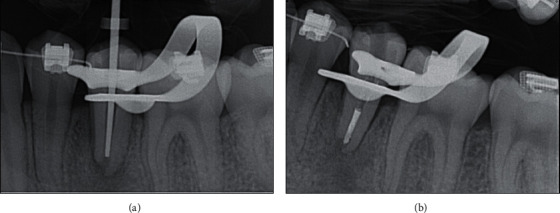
Obturation radiographs. (a) Periapical radiograph of endodontic plugger measurement of tooth #20. (b) Periapical radiograph of 4 mm apical plug of tooth #20.
